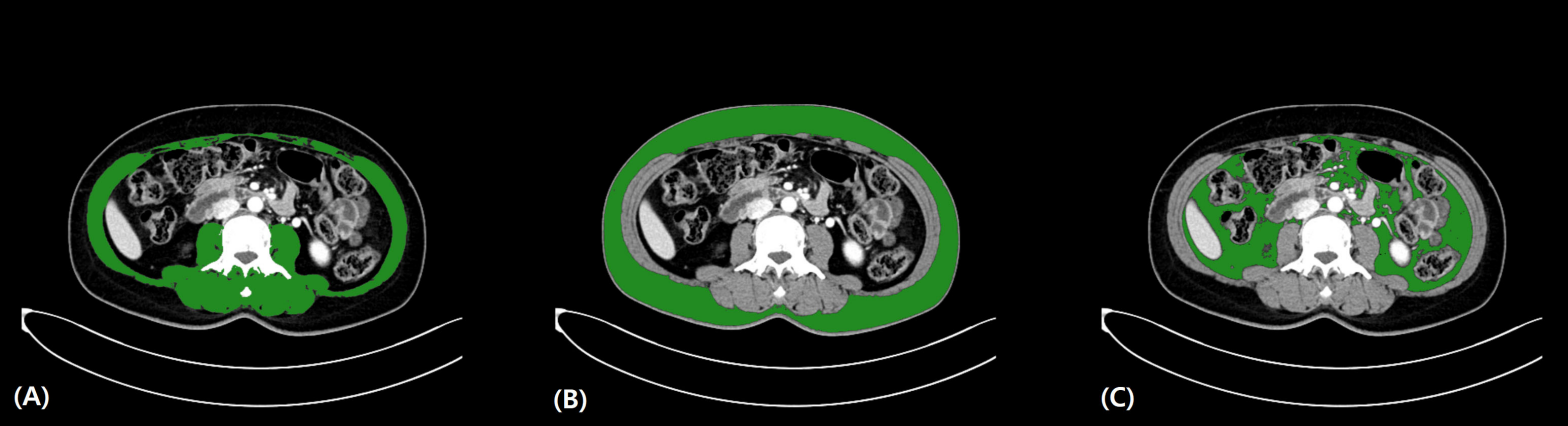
Extracted CT axial cut at the level of the third lumbar vertebra of the (A) skeletal muscle, (B) subcutaneous fat, and (C) visceral fat areas. The HU range was -29 to 150 HU for skeletal muscle tissue, -190 to 30 HU for subcutaneous adipose tissue, and -50 to 150 HU for visceral adipose tissue. CT, computed tomography; HU, Hounsfield unit.
